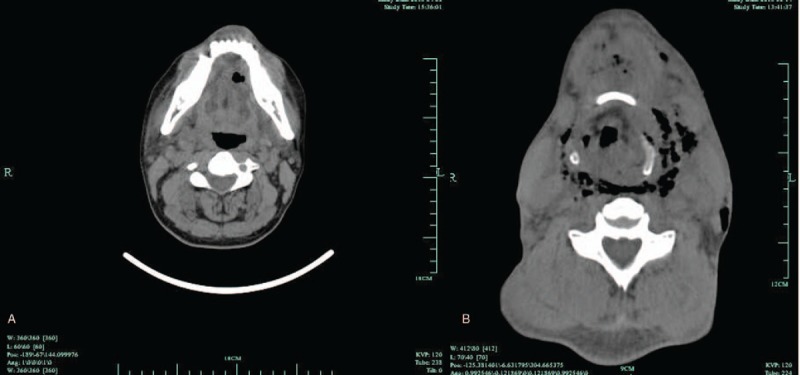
(A) Computed tomography (CT) scanning showed the formation of a left sublingual abscess following first molar periodontitis. (B) CT scanning showed abscess formation and gas production in parapharyngeal and submental spaces.
